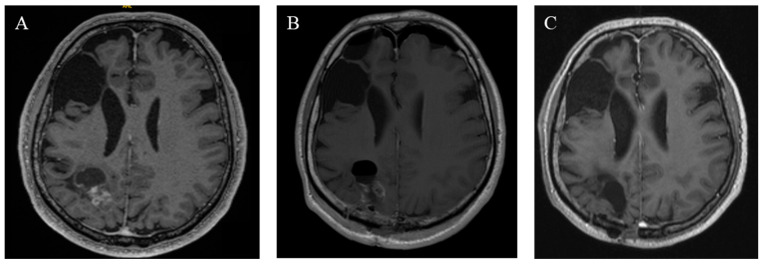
Radiographic findings of case 11. (A) Preoperative T1 MRI with a contrast agent. (B) Postoperative MRI on the first postoperative day. (C) Three years of follow-up MRT after adjuvant radiation.
